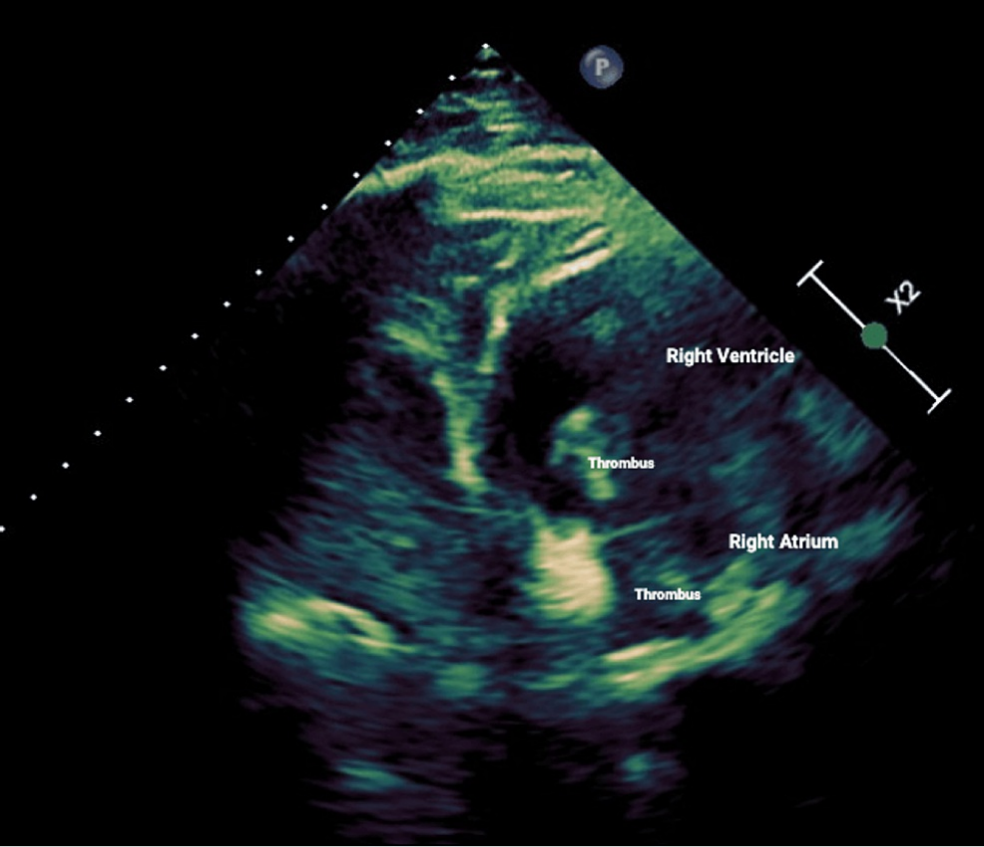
2D echocardiogram view showing intracardiac thrombus extending within the right atrium and right ventricle
